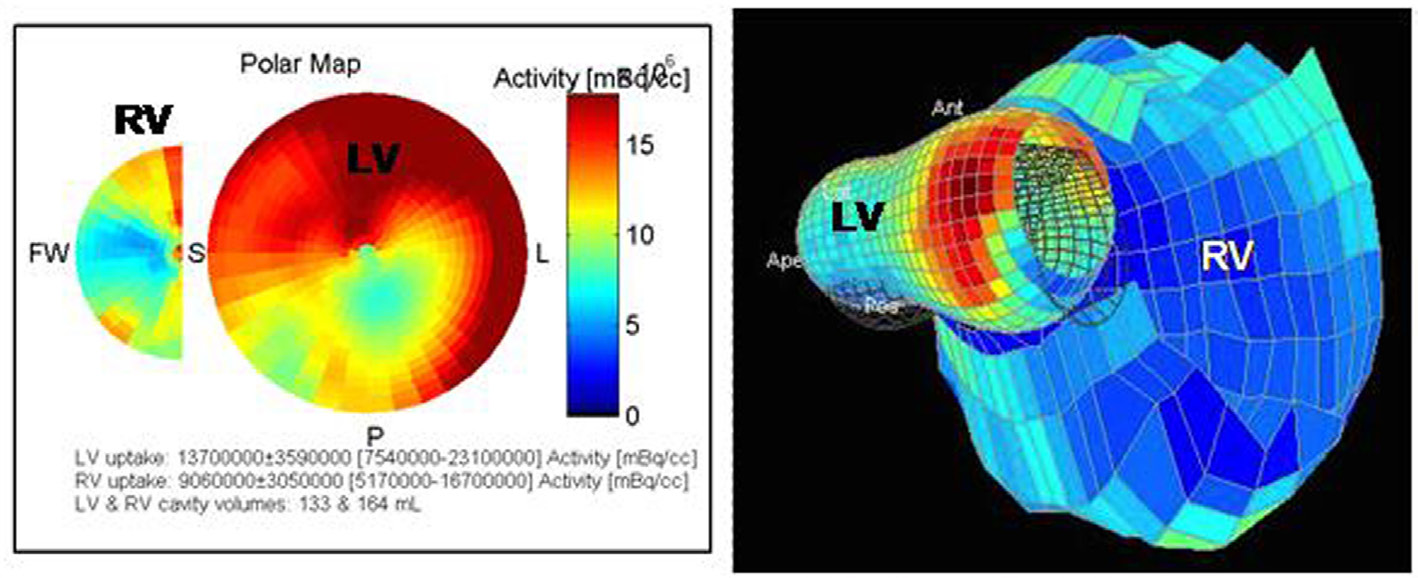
Pictures obtained from a 67-year-old man with PH of mixed origin (COPD, left heart dysfunction). 18F-FDG-PET/CT shows a dilation of the RV with an increased and heterogeneous uptake. A hypometabolism of the infero-lateral medial wall is found in the LV (own unpublished data).
